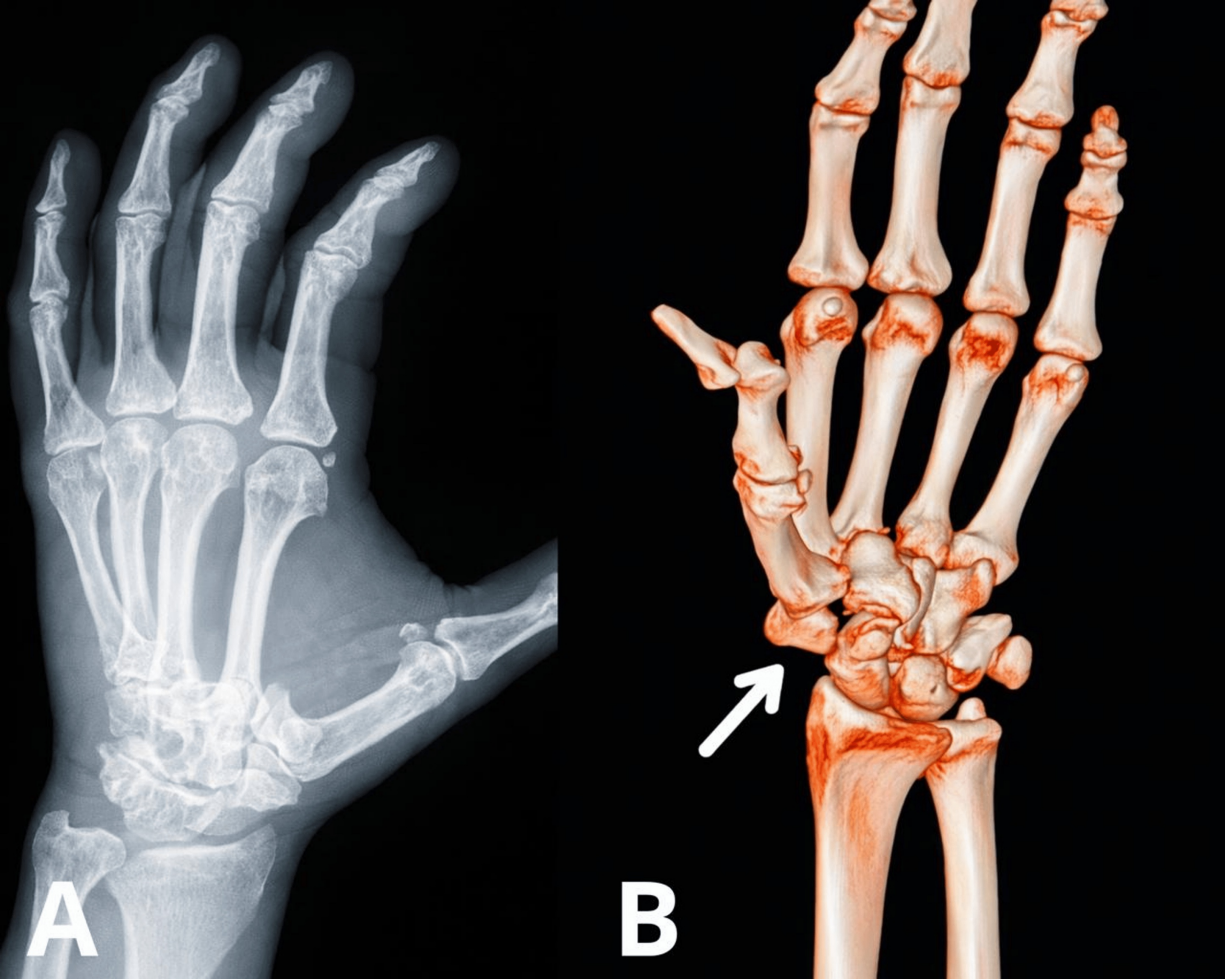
Imaging studies demonstrating trapeziometacarpal dislocation (A) AP radiograph of the hand. (B) CT scan with 3D reconstruction.
